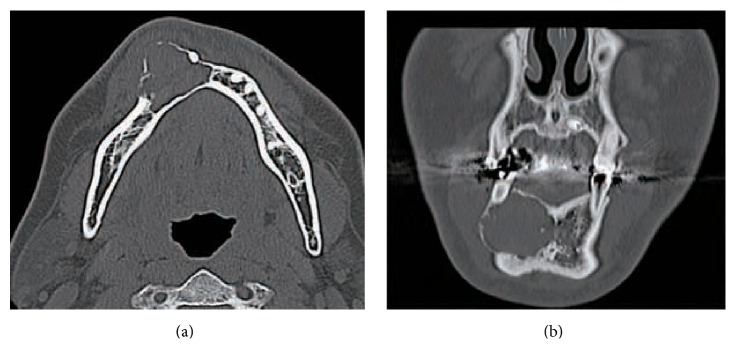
(a) Axial and (b) coronal computed tomography scans showing a large unilocular radiolucent lesion in the right mandible.
